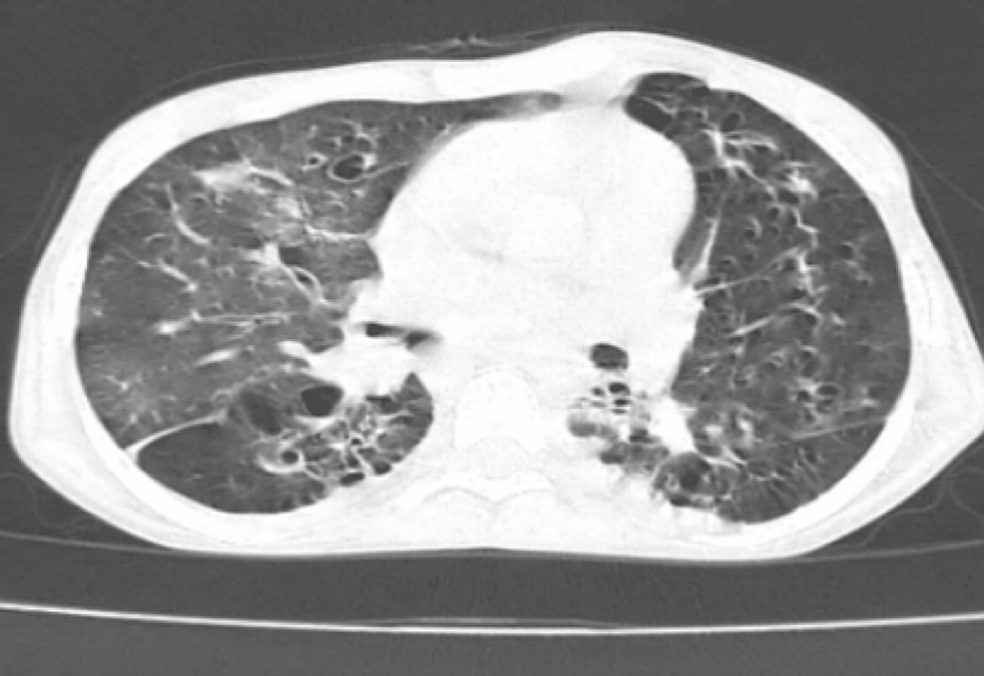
CT of the thorax showing co-existing emphysematous hyperinflation
